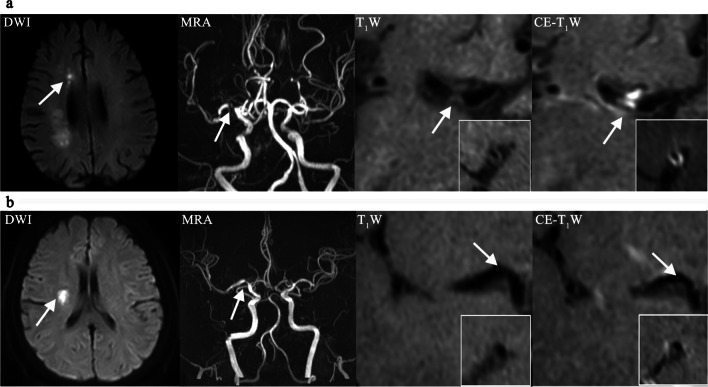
Example images of patients with and without stroke recurrence. a. A patient with stroke recurrence. DWI shows infarct in the right corona radiata (arrow). MRA shows severe stenosis on the M1 segment of right MCA (arrow). Pre- (T_1_W) and post-contrast (CE-T_1_W) HR-MRI detect a plaque (arrow) with higher plaque burden (89.8%) and enhancement ratio (0.84). b. A patient without stroke recurrence. DWI shows infarct in the right corona radiata (arrow). MRA shows moderate stenosis on the M1 segment of right MCA (arrow). Pre- (T_1_W) and post-contrast (CE-T_1_W) HR-MRI detect a plaque (arrow) with smaller plaque burden (81.5%) and enhancement ratio (0.27). DWI: diffusion weighted imaging; MRA: magnetic resonance angiography; MCA: middle cerebral artery
